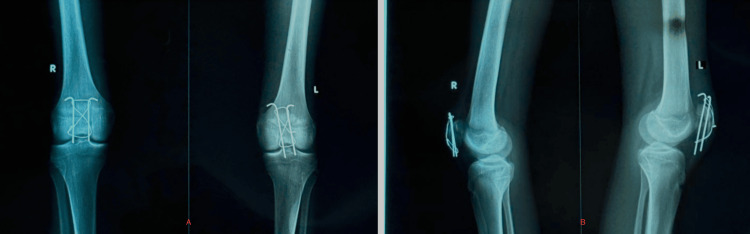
Postoperative anteroposterior radiographs of both knees confirming anatomical reduction and satisfactory bilateral tension-band wiring fixation of the patellae. The right knee (R) and left knee (L) are labeled accordingly.
